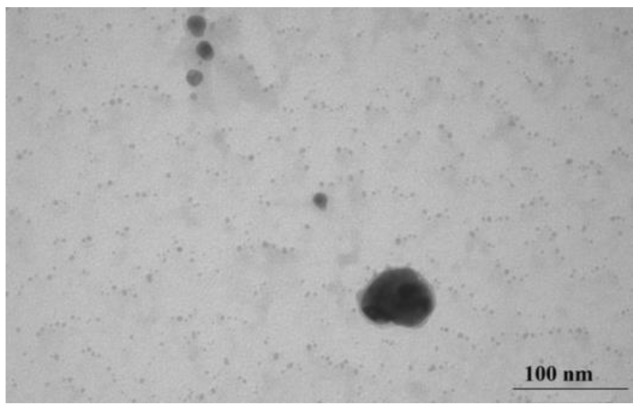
TEM image of the product of thermolysis of silver fumarate in an aqueous medium by ultrasound after 20 min of treatment.
